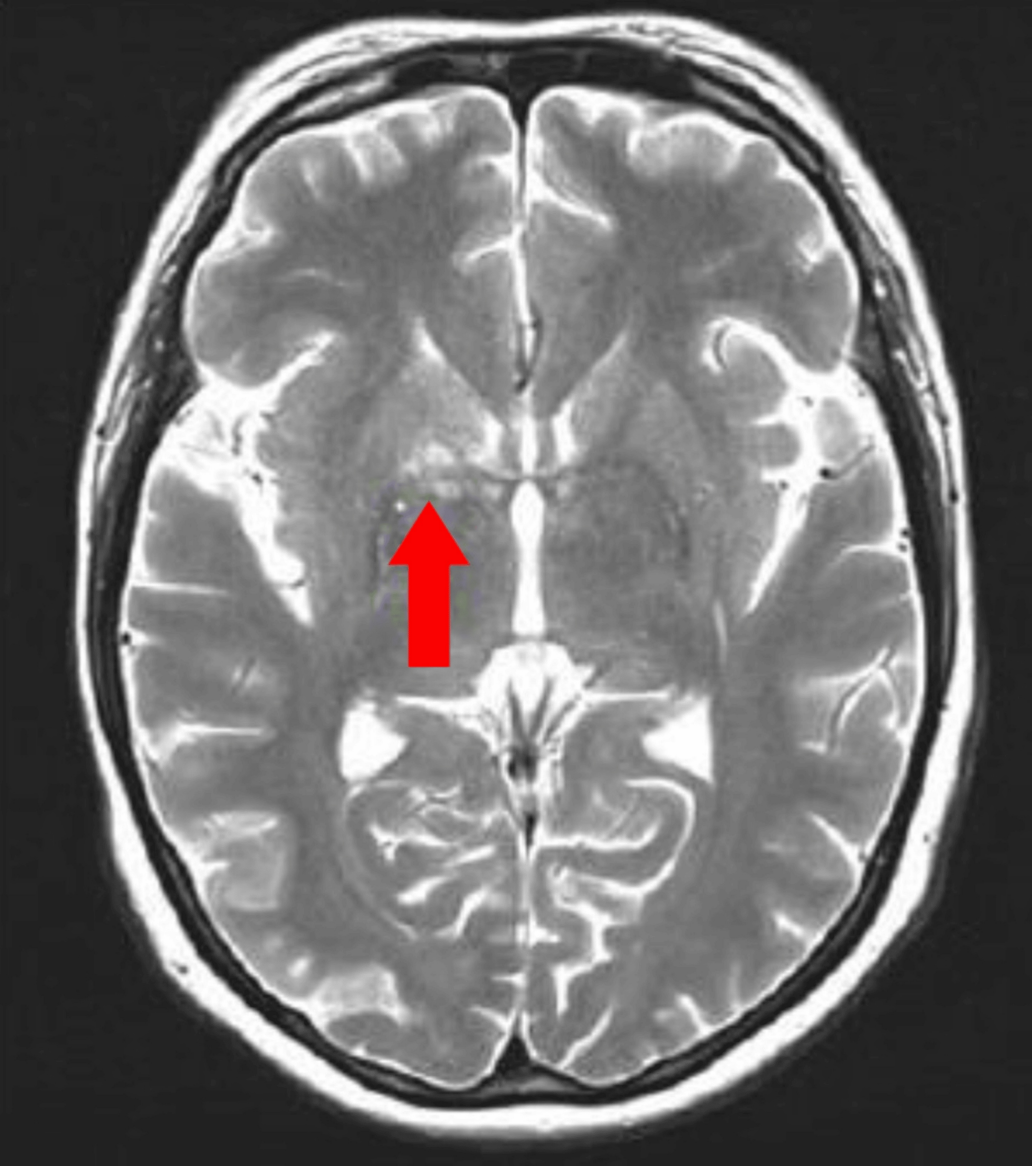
T2-weighted MRI of the brain shows hyperintense lesions at the right globus pallidus (red arrow).
